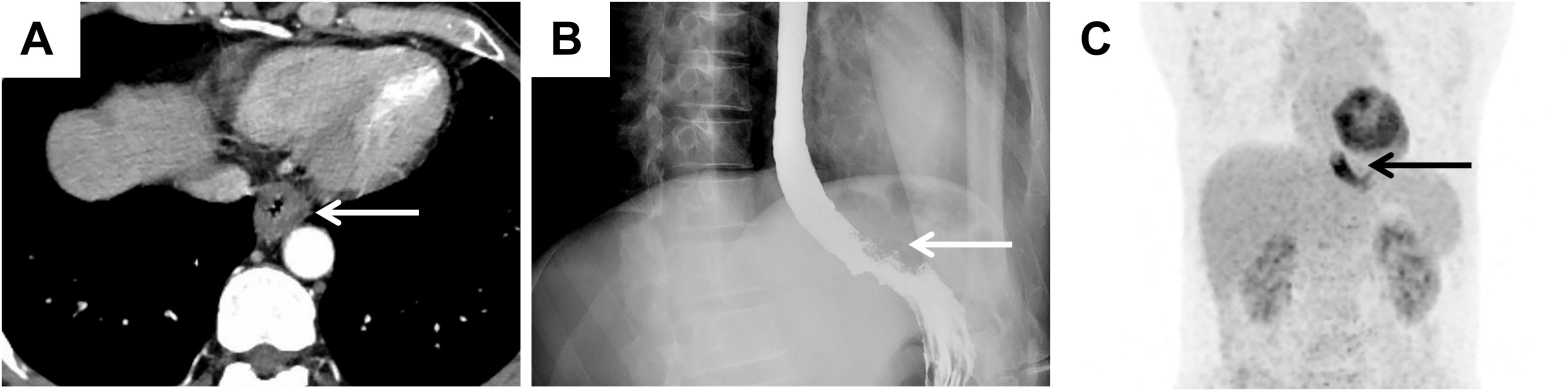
Imaging findings suggested the possibility of malignancy in the oesophagus. (A) CT image suggesting significant thickening of the wall of the lower oesophagus at the gastroesophageal junction (white arrow). (B) The results of oesophagography revealed narrowing of the distal oesophageal lumen (white arrow). (C) PET–CT image showing significant thickening of the wall of the lower thoracic oesophagus with increased glucose metabolism (black arrow).
